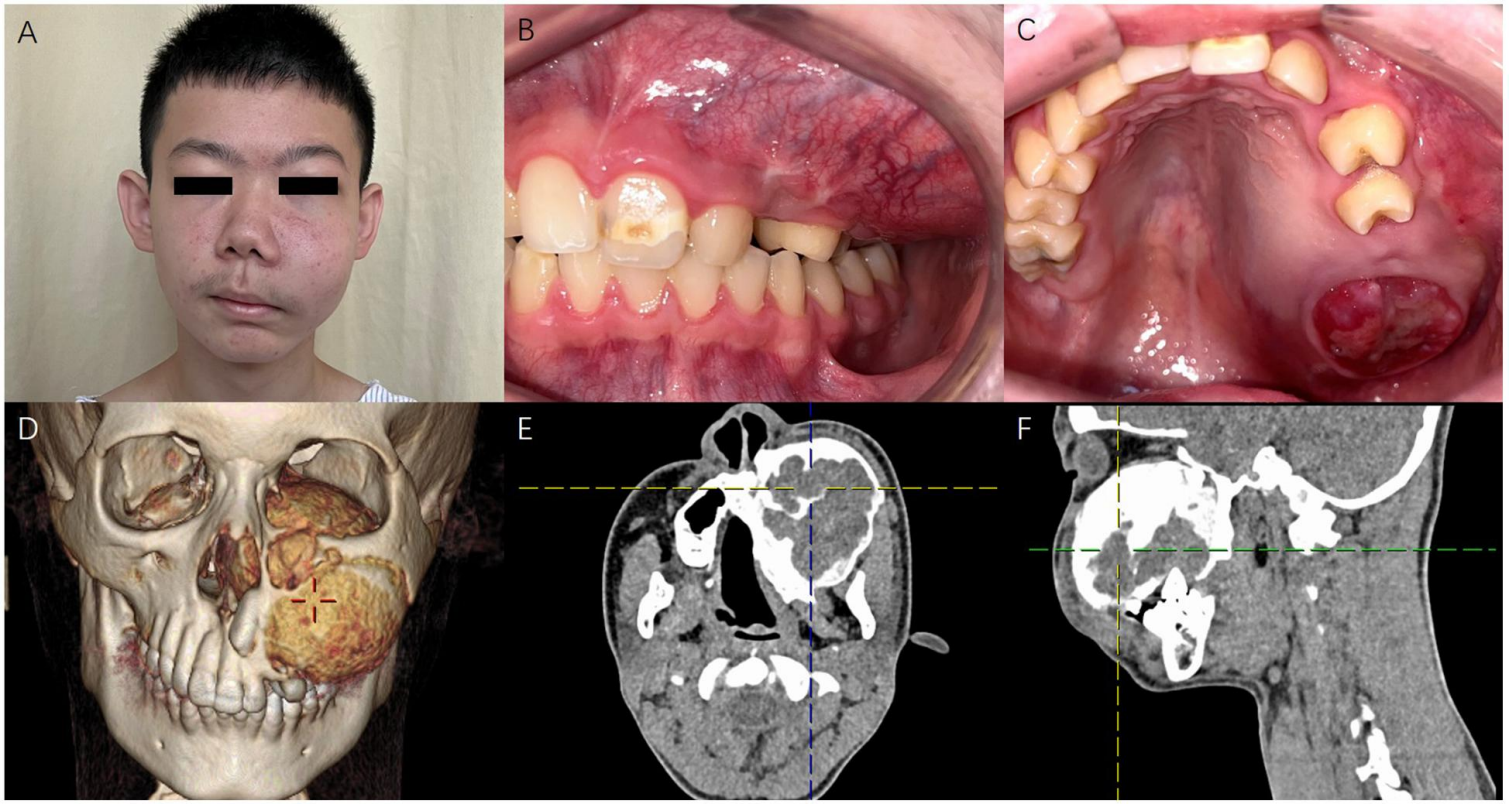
(A), photograph of the patient displaying facial asymmetry due to left maxillary tumor. (B,C), intraoral examination showing left maxillary mass. (D–F) maxillofacial CT revealed a 7.7 × 6.0 × 6.0 cm mass arising from the left maxilla, filling the left maxillary sinus. The mass was heterogeneous with mixed areas of density, with a clear and uneven thickness margin.
